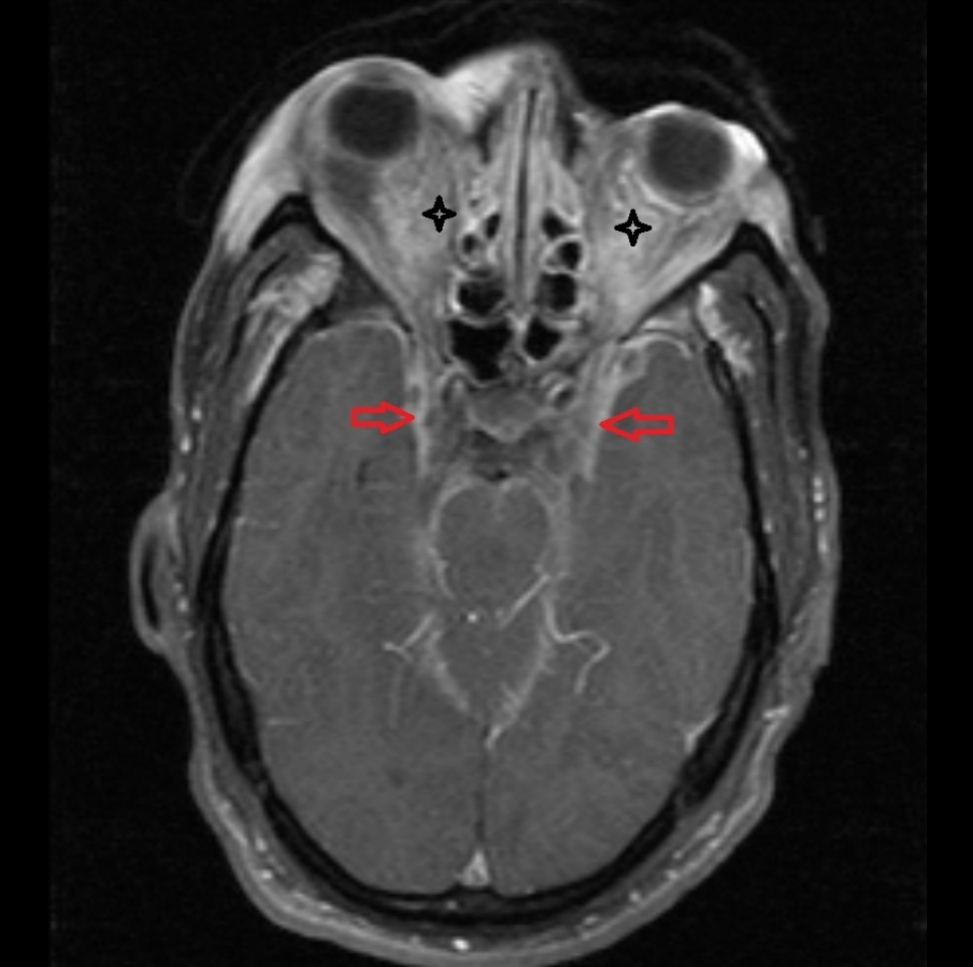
Magnetic resonance imaging (MRI) of the brain showing extensive inflammatory/infectious changes involving the intraconal and extraconal fat of the right and left orbits (black stars) concerning for orbital cellulitis. Meningeal enhancement is also seen at the temporal regions concerning for meningitis (read arrows)
